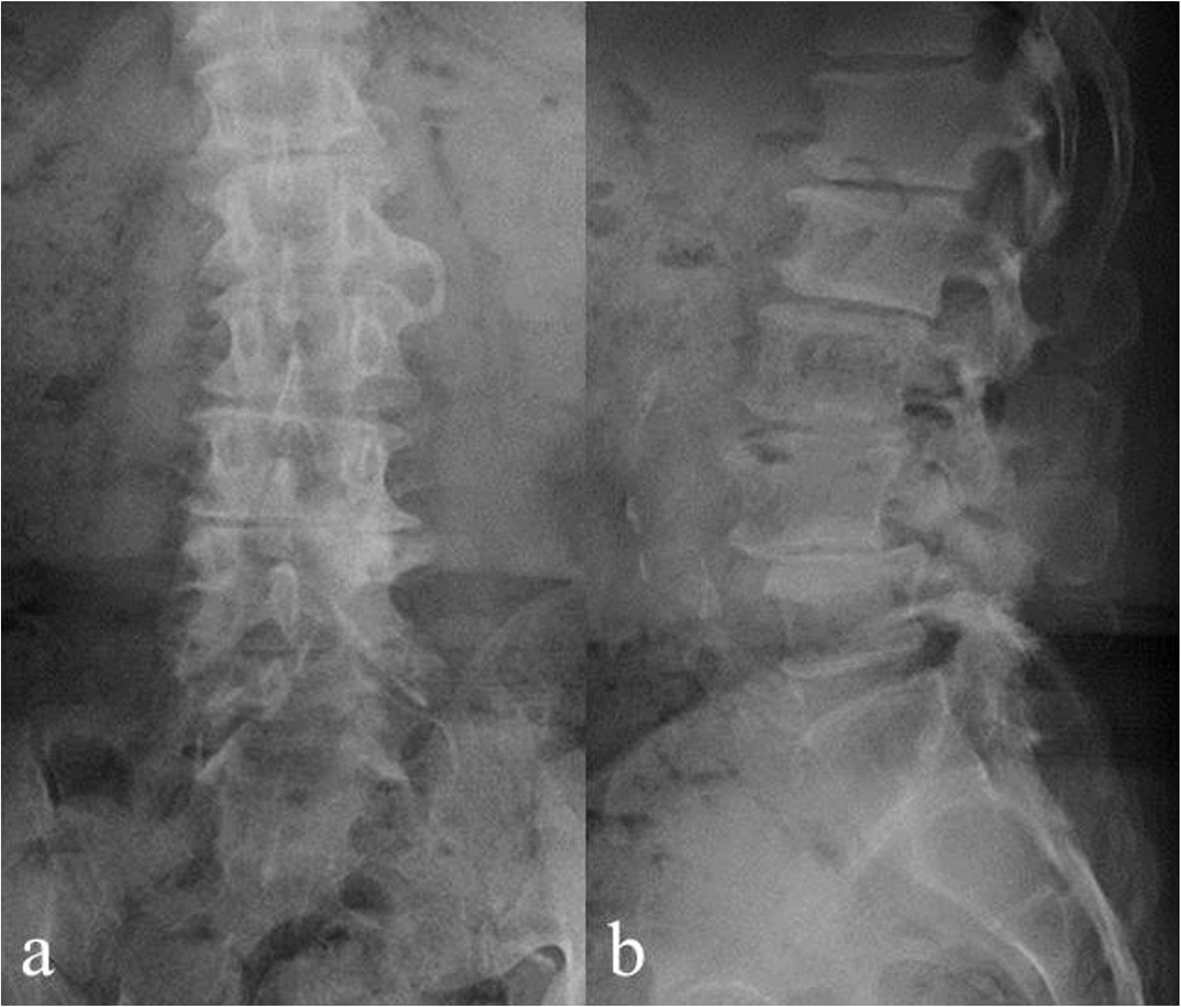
Preoperative radiographic imaging. Preoperative radiographic coronal image a and radiographic lateral image b showing Meyerding grade II spondylolisthesis
